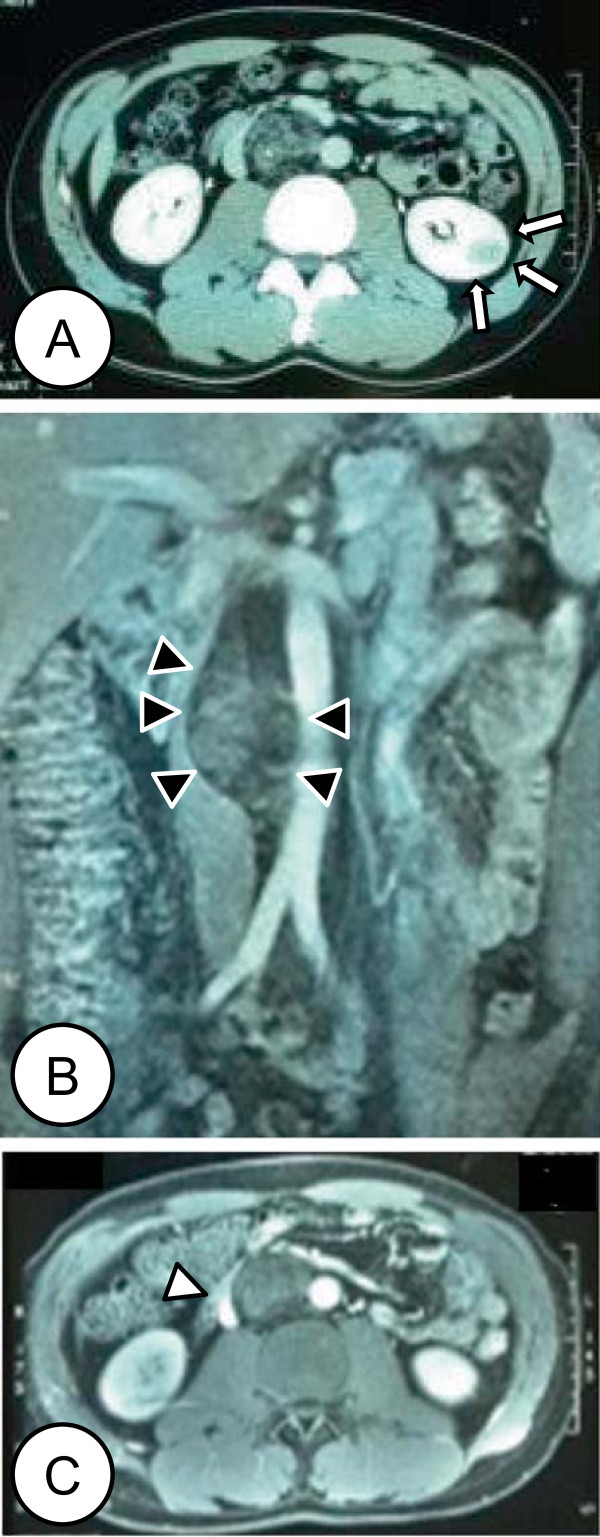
Comuted tomography (CT) and magnetic resonance imaging (MRI) of the tumors. Left lower pole renal tumor (indicated by arrows) and 5x4x7.5 cm interaortocaval tumor containing fatty tissue, calcification and soft tissue components (indicated by filled arrowheads). The inferior vena cava is compressed by the ineraortocaval tumor (indicated by open arrowhead). (A) Contrast-enhanced CT, parenchymal phase. Wash-out of the contrast is typical for clear cell RCC. Coronal (B) and axial (C) contrast-enhanced MRI.
